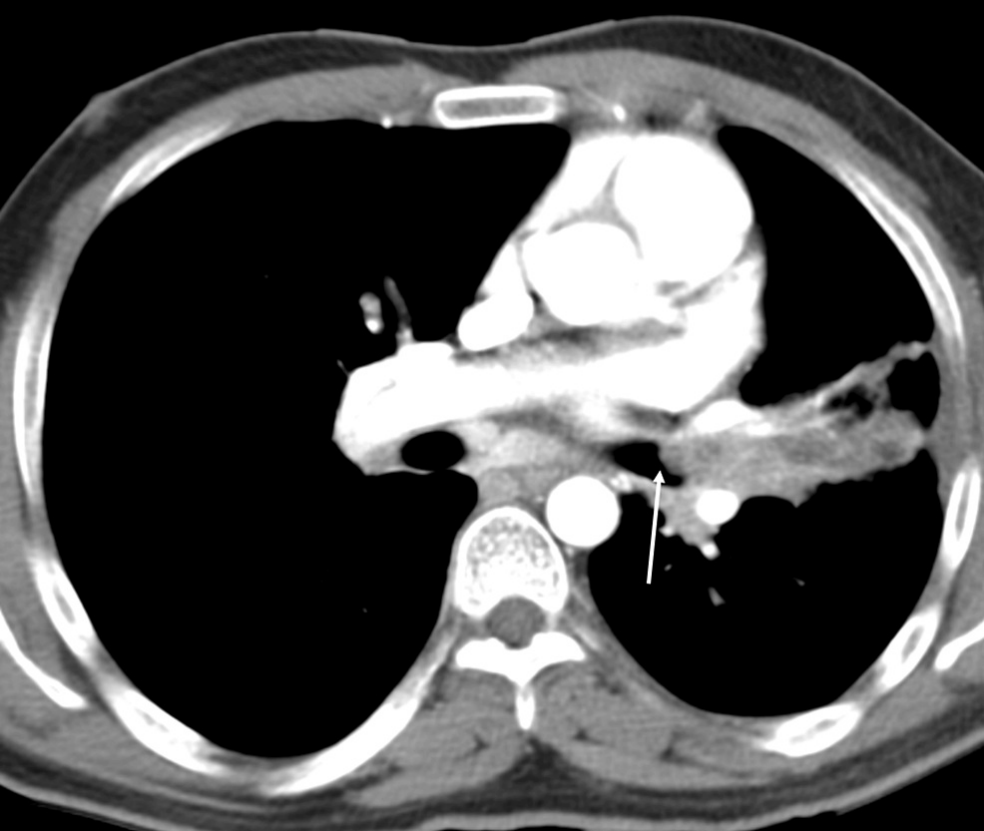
CECT showing volume loss in the LUL, endobronchial LUL lesion, mediastinal lymphadenopathy, and poorly defined low-density pulmonary nodules (October 21, 2011) CECT: contrast-enhanced computed tomography; LUL: left upper lobe
